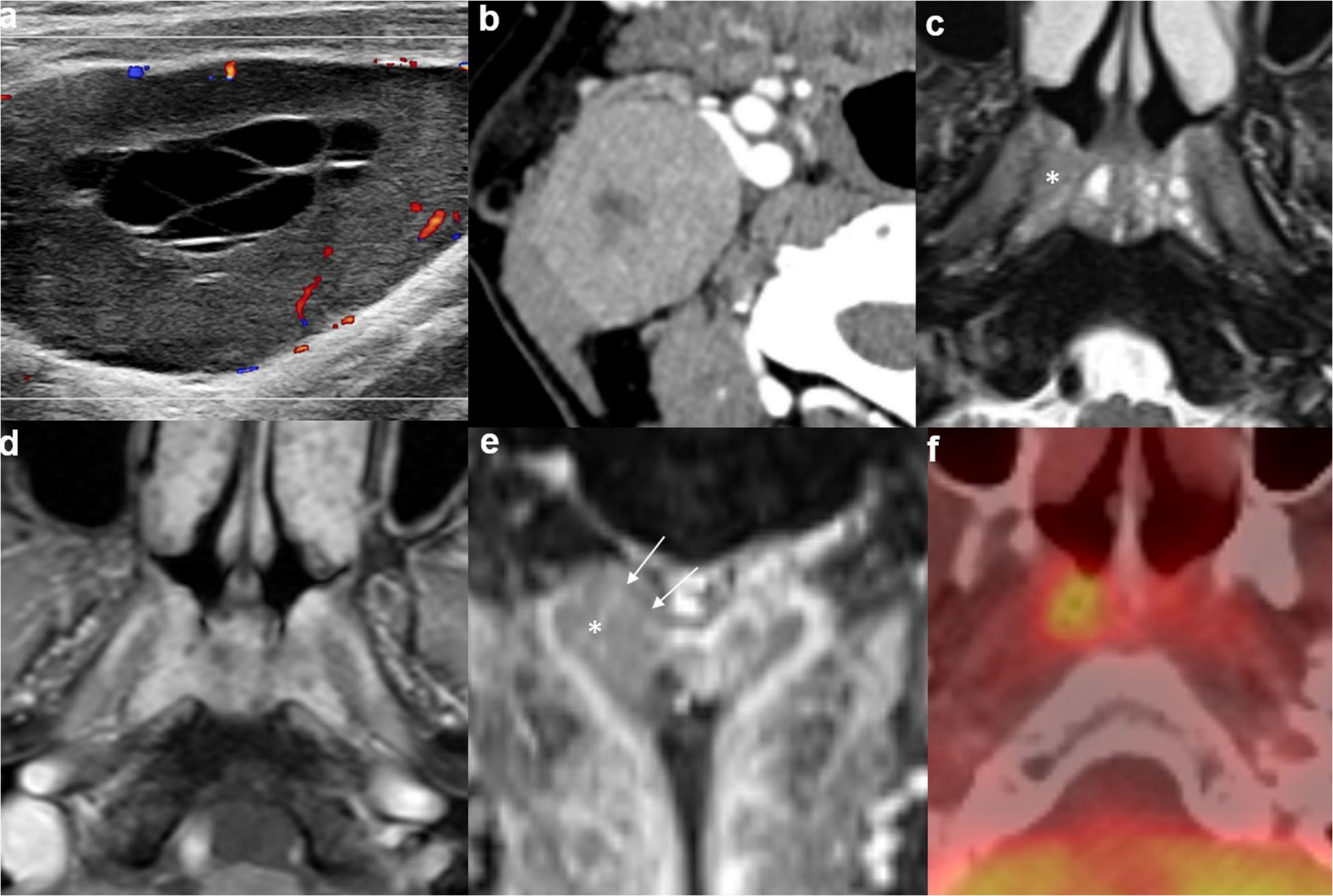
Coexistence of nasopharyngeal tumor and lymphoid hyperplasia in a 70-year-old man referred for an enlarging right neck mass. Doppler ultrasound of the right neck shows a hypoechoic mass with increased peripheral vascular flow and a central anechoic cystic area with septations (a). Axial contrast CT of the neck shows an enlarged roundish right level II node with central hypoenhancing area suggestive of necrosis (b). Prominent soft tissue density in the nasopharynx was noted on the CT (not shown). Endoscopy found a right nasopharyngeal mass and biopsy was proven to be an undifferentiated carcinoma. Axial T2-weighted image shows soft tissue thickening of the bilateral nasopharynx. Small cysts are seen involving the bilateral nasopharynx, more on the left side. Minimal intermediate T2-weighted soft tissue thickening is seen at the lateral wall of right nasopharynx (asterisk) (c). The pharyngobasilar fascia is intact. Axial post-contrast T1-weighted, fat-suppressed image shows only non-specific moderately enhancing soft tissue thickening of the bilateral nasopharynx with intact deep mucosal white line (d). Coronal post-contrast, fat-suppressed T1-weighted image depicts a moderately enhancing mass in the right nasopharynx (asterisk) with focal loss of the deep mucosal white line at the roof (arrows) (e). Axial fusion FDG-PET/CT depicts the hyper-metabolic right nasopharyngeal mass and the hypermetabolic right level II node (not shown). Otherwise, no hypermetabolic distant metastasis (f). Radiological staging was T1N1M0 and the patient received radical radiotherapy. This case highlights three diagnostic pearls. First, the presence of benign lymphoid hyperplasia and tumor are not mutually exclusive. Second, the use of multiplanar reformat should be routinely performed for evaluation of the nasopharynx in order to avoid missing small tumors due to partial volume effect. Third, level II nodal metastasis can occur without involvement of the retropharyngeal node, especially for laterally located tumors in the nasopharynx
